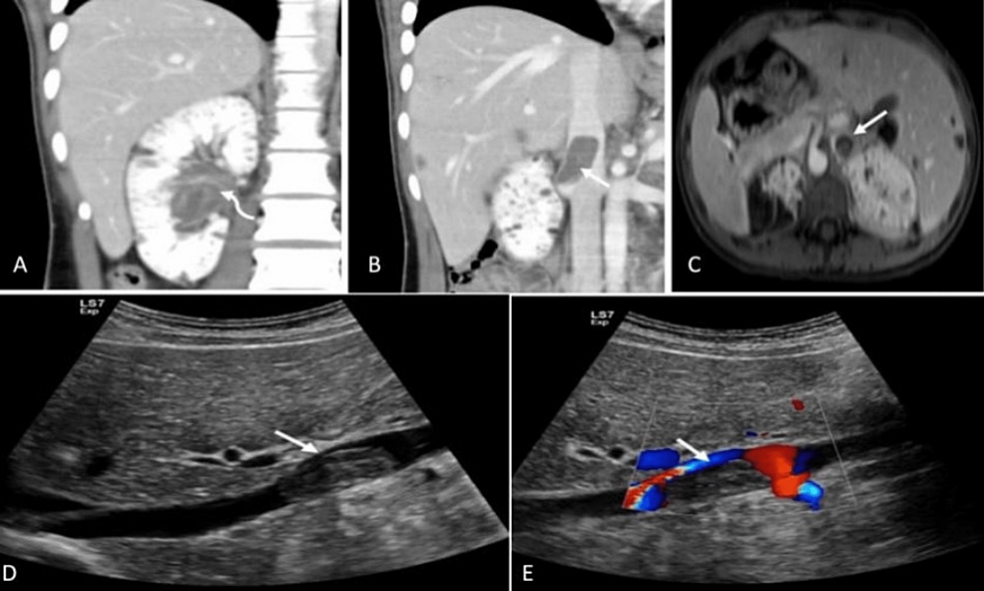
Contrast-enhanced CT scan of the abdomen and pelvis (a, b) Coronal portovenous contrast enhanced abdominal CT scan, (c) Axial T1-weighted VIBE sequence fat-saturated post contrast, (d) Grey scale and (e) color Doppler ultrasound images demonstrate a heterogeneous mass (curved arrow) in the right renal pelvis (a) with an apparent direct extension through the right renal vein into the IVC (b, c, d) with no internal vascularity (e) (arrow).
